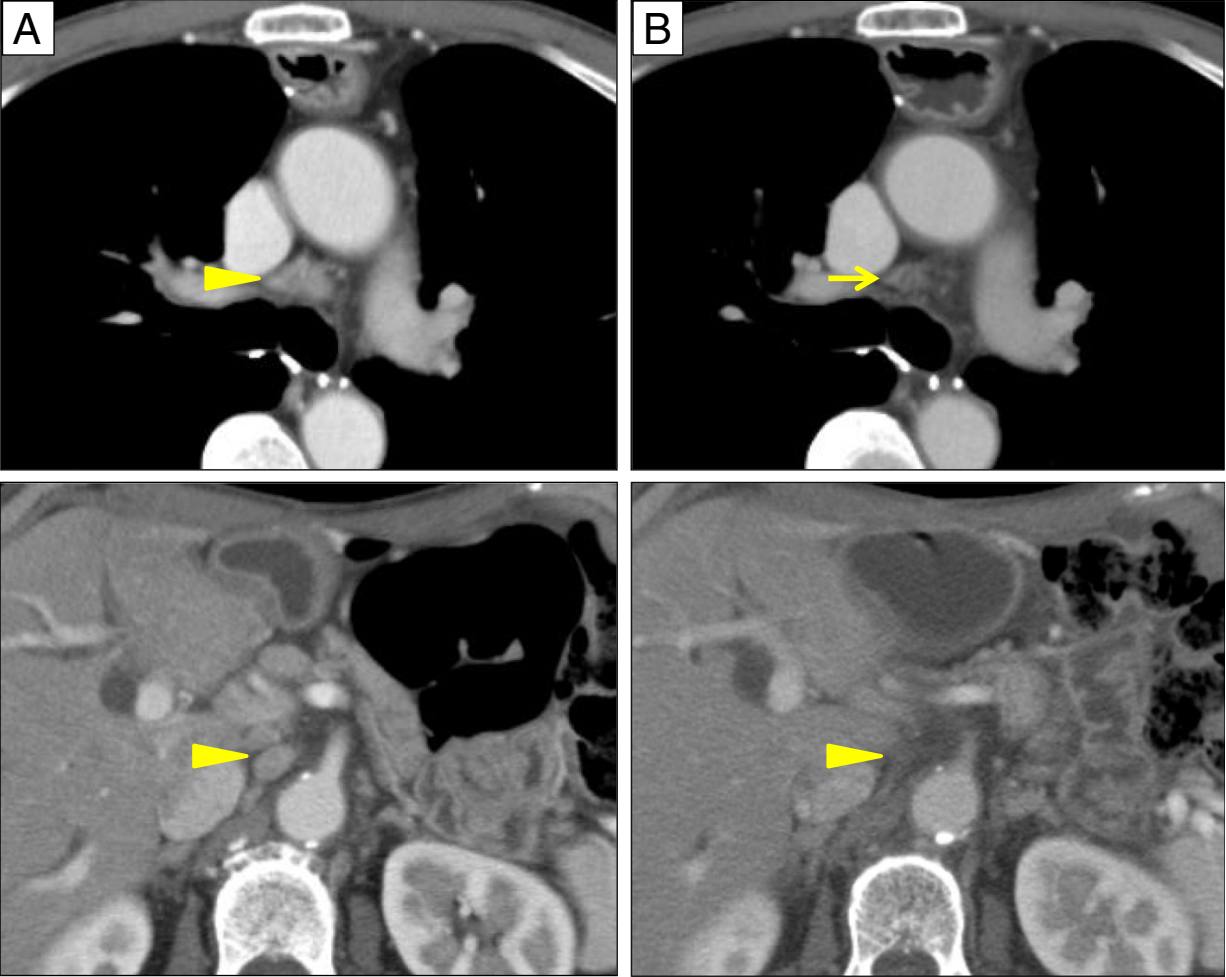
CT images showing metastases in multiple mediastinal and intra-abdominal LN. a Before chemoradiation (arrowhead). b After treatment, showing mediastinal LNs decreased and intra-abdominal LN disappeared (arrowhead)
